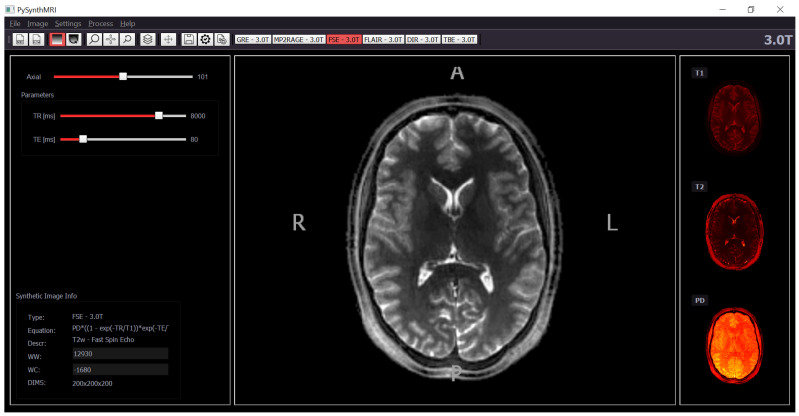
The graphical interface of PySynthMRI. On the left, the provided scanner parameter. In the middle, the synthesized FLAIR image. On the right, the input quantitative maps.
